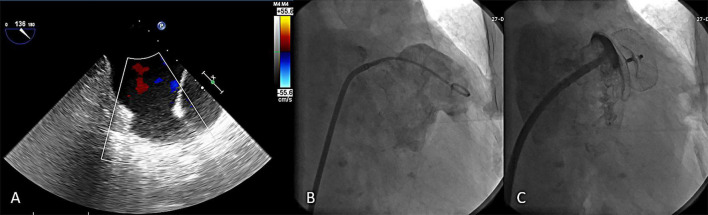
The largest LACbes device (34 mm) in a shallow left atrial appendage. (A) TEE in 135° view showed a large and shallow LAA; (B) LAA angiography in a RAO 30° + caudal 20° projections showed a “chicken wing” LAA; (C) good sealing after LACbes device implantation under LAA angiography. LAA, left atrial appendage; TEE, transesophageal echocardiography.
